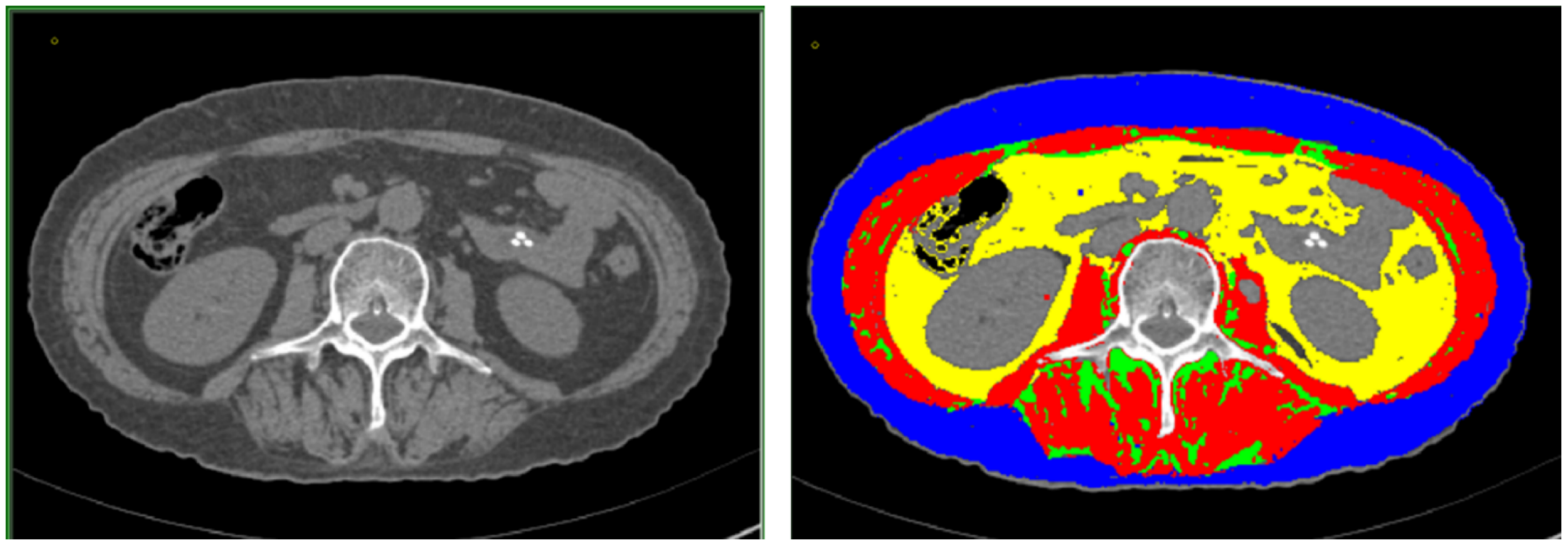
(Left) Cross-sectional image of the third lumbar vertebra acquired using computed tomography. (Right) sliceOmatic image of muscle fat composition at the third lumbar vertebra: visceral adipose tissue, intramuscular adipose tissue, subcutaneous adipose tissue, and skeletal muscle are marked by the yellow, green, blue, and red regions, respectively.
